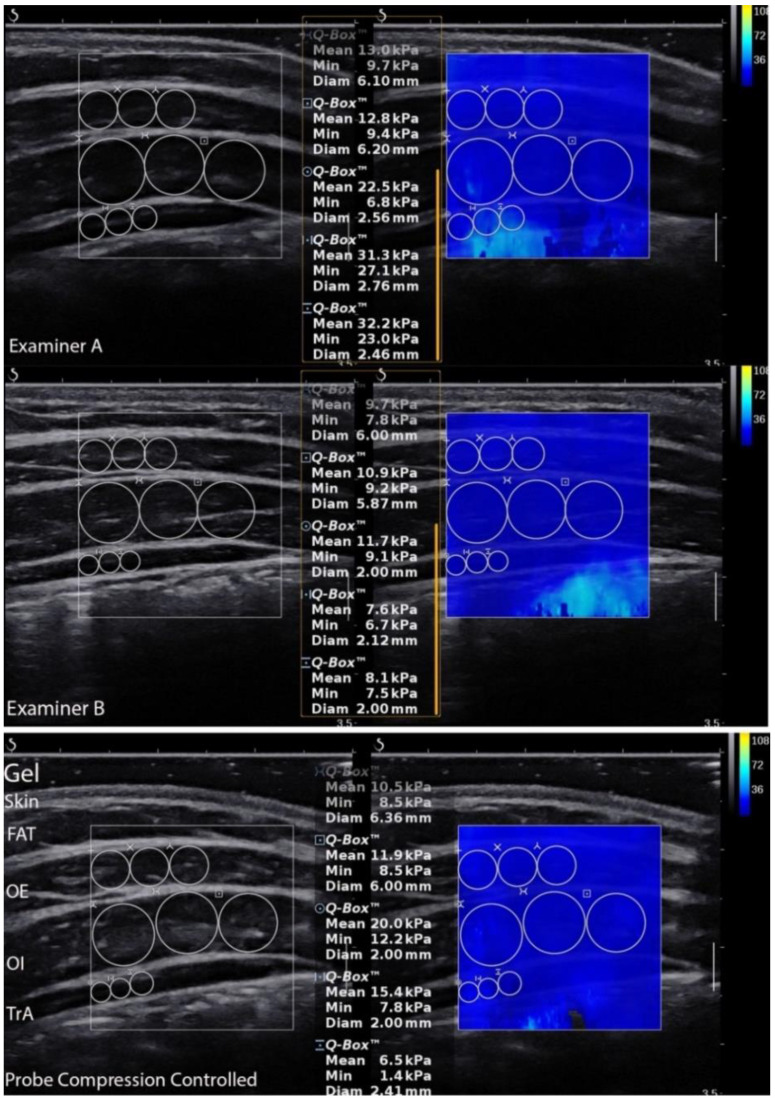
Ultrasound scans of the lateral abdominal muscles performed by all examiners and shear modulus measurements. FAT—superficial fat layer; OE—oblique external; OI—oblique internal; TrA—transversus abdominis muscle.
